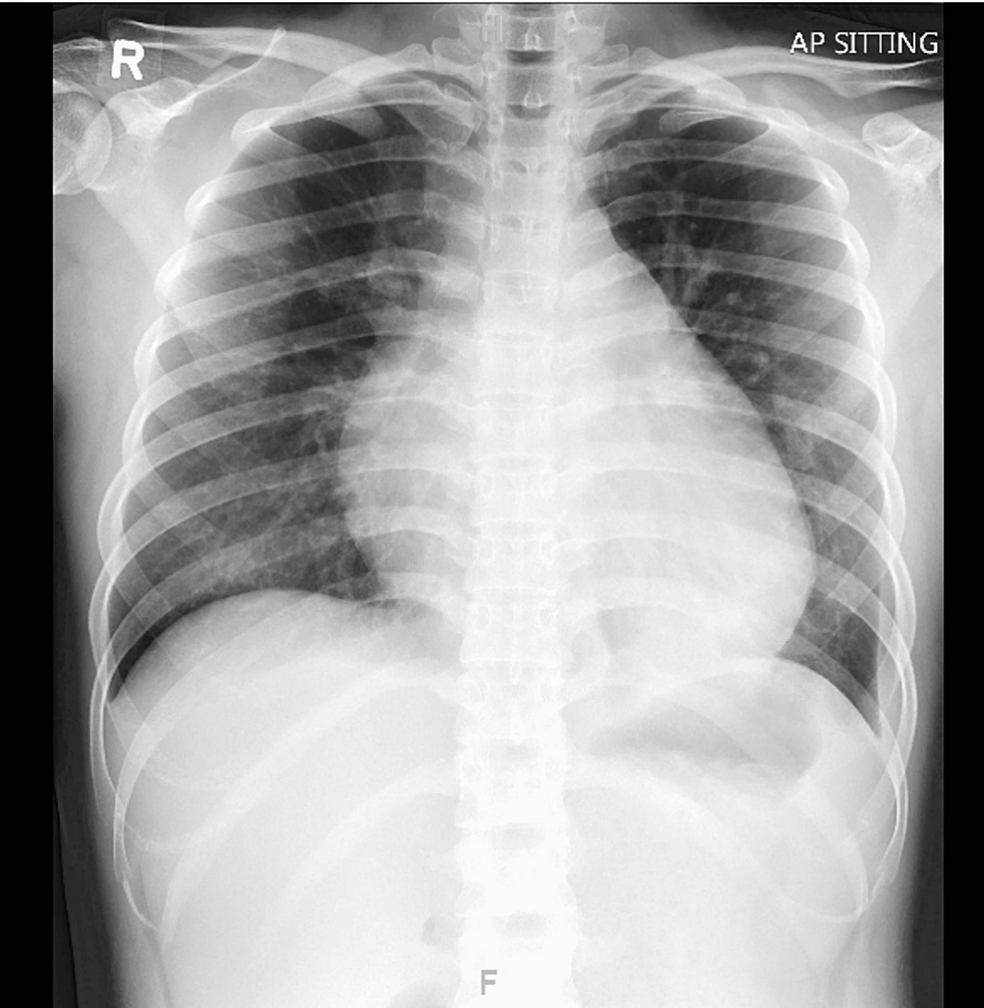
Chest X-ray showing that the heart is moderately enlarged and globular with prominent vascular markings
